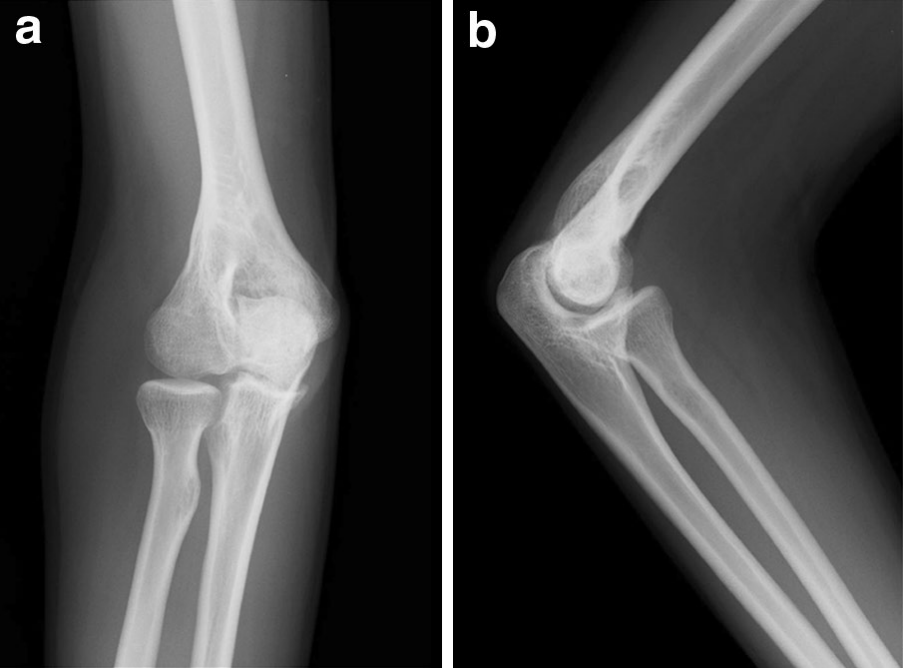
Plain radiography findings 1 year after curettage and artificial bone grafting. One year after curettage and artificial bone grafting, plain radiography of antero-posterior (a) and lateral (b) views show no recurrence. The patient returned to daily activities without any symptoms.
